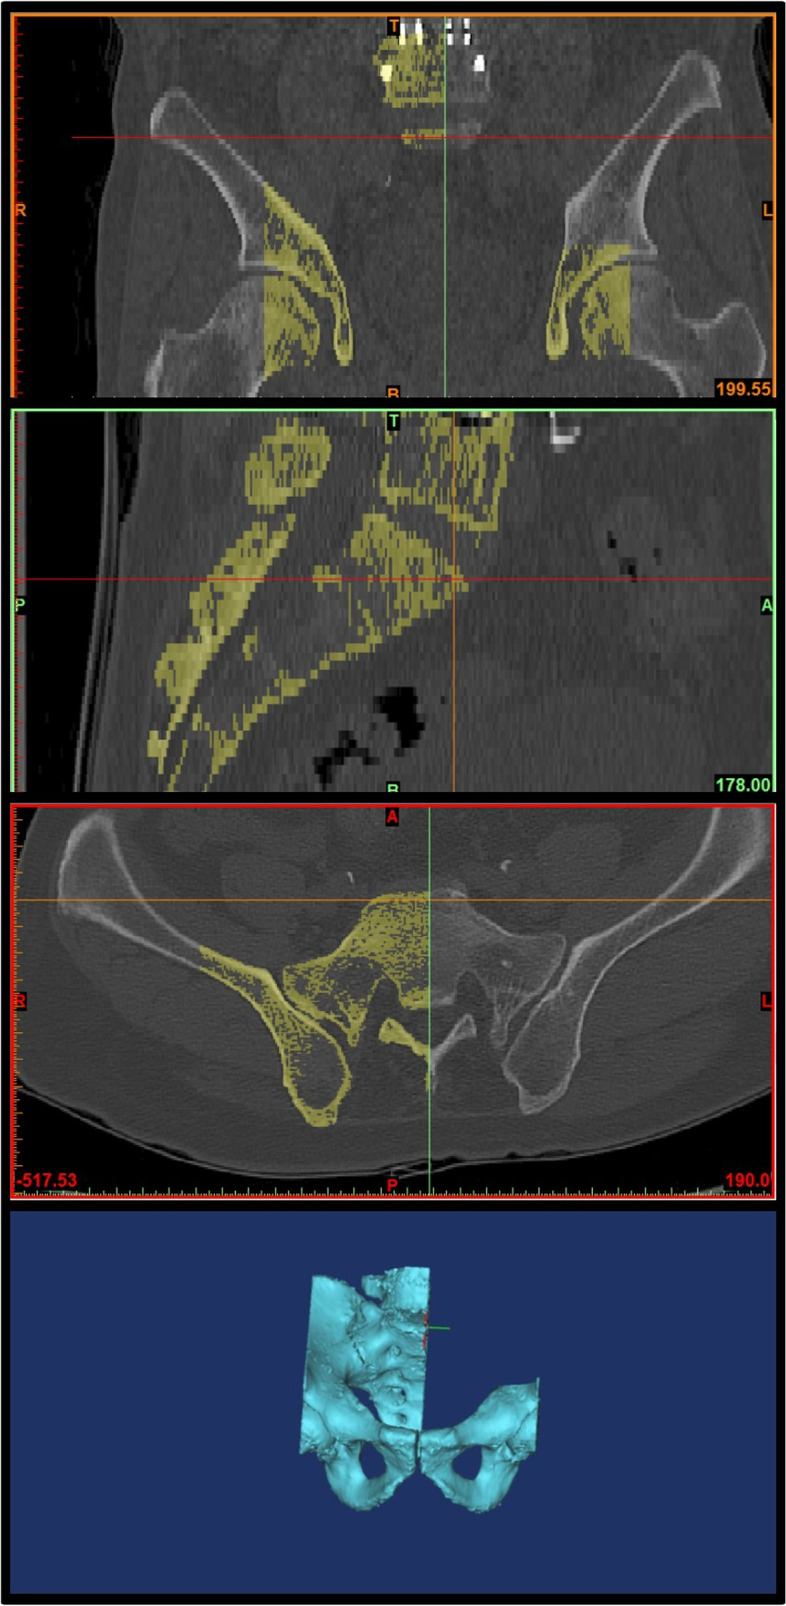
3D reconstruction of sacrum and lower lumbar for pelvic incidence; Identification of S1 end plate of CT scans and segmentation of S1 endplate in coronal, sagittal, and axial plane. Remove left sacrum and lower lumbar images
